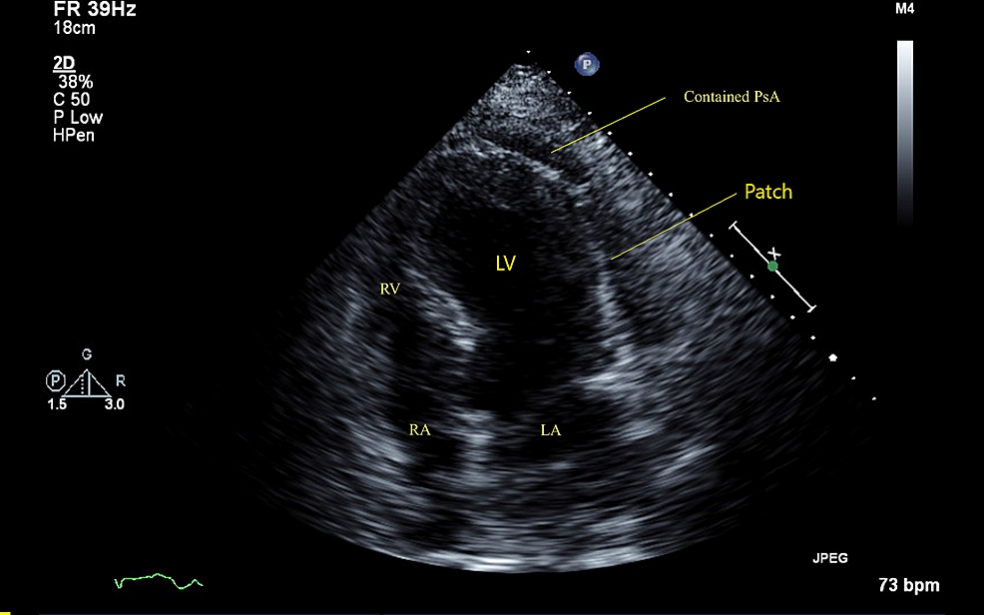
2D-TTE (apical four-chamber view) The image shows contained pseudoaneurysm of LV lateral wall consistent with Hemashield patch repair. 2D-TTE: Two-dimensional transthoracic echocardiography, LA: left atrium, LV: left ventricle, RA: right atrium, RV: right ventricle, PsA: Pseudoaneurysm
